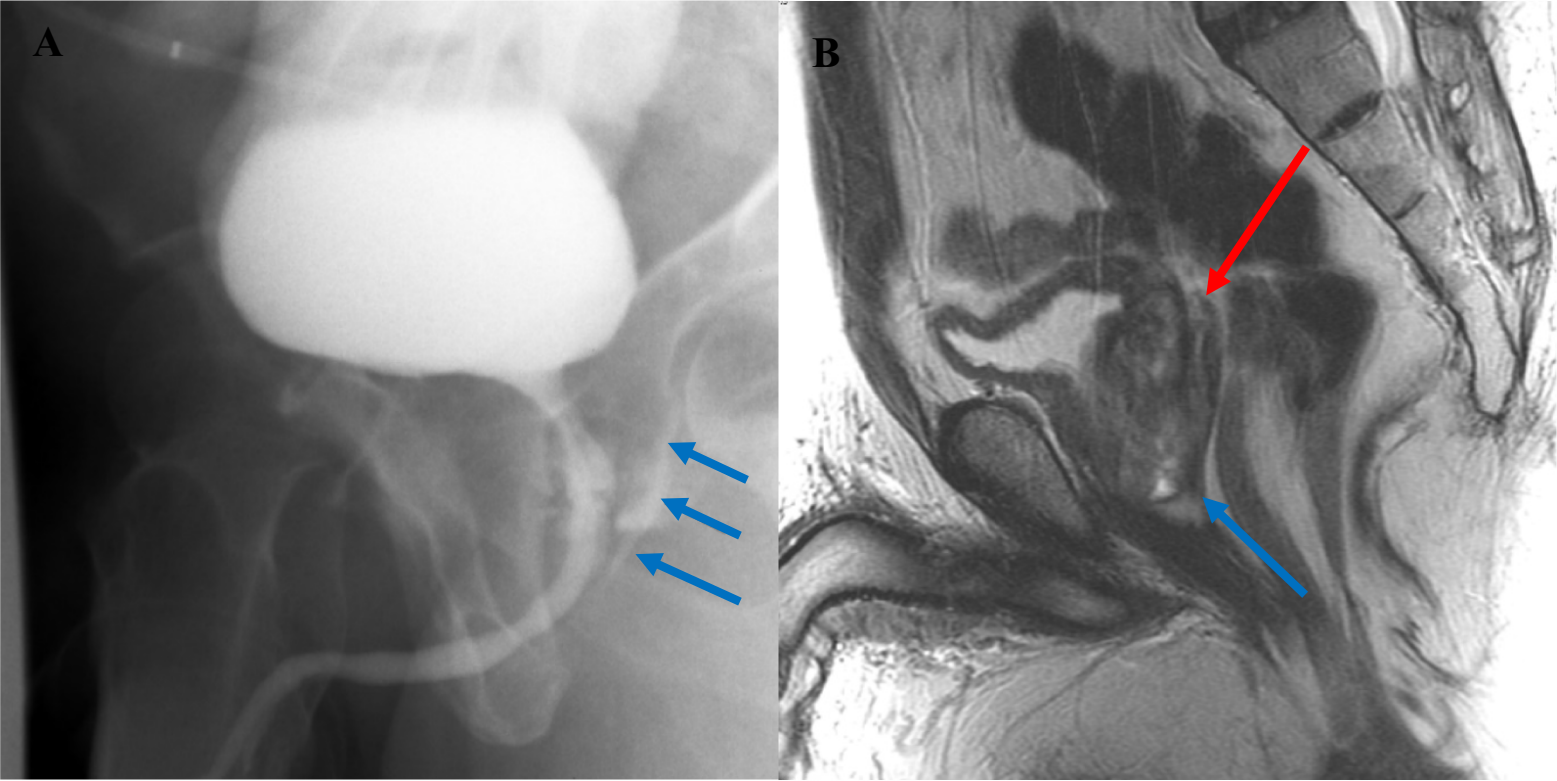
A Postoperative voiding cystourethrogram shows small sinus tract posterior to urethra (arrows), but no fistula to rectum. B Postoperative T2-weighted MRI shows resection of the cystic structure. Linear structure posterior to prostate corresponds to sinus tract on cystourethrogram (red arrow at superior border, blue arrow at most distal border). No residual abscess was seen
